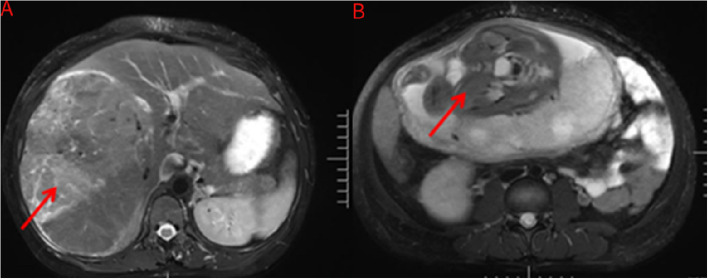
A Abdominal magnetic resonance imaging revealed a space occupying lesion of the right liver. B Fetal MRI was normal
